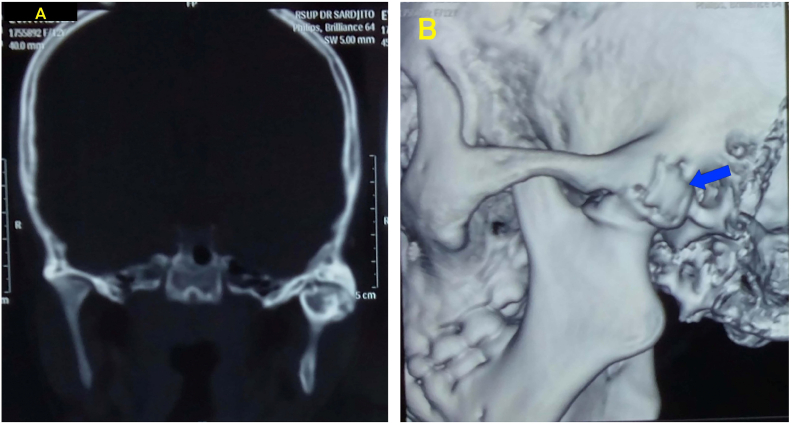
A and B Preoperative CT and 3D CT image before the first surgery, showed lateral and medial extensions of the ankylotic mass of left TMJ ankylosis (blue arrow). (For interpretation of the references to colour in this figure legend, the reader is referred to the web version of this article.)
